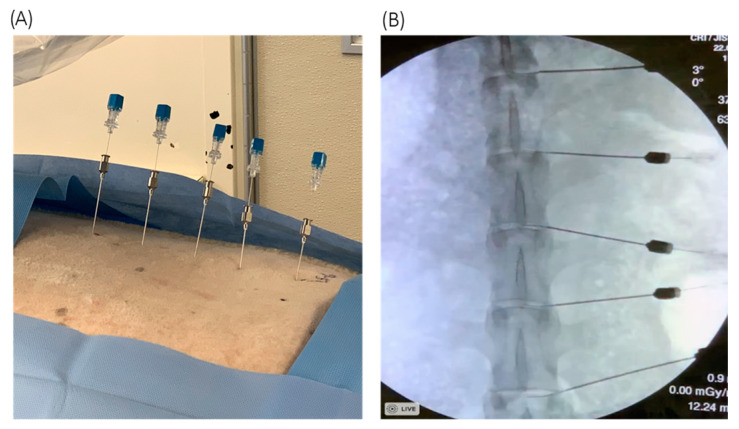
(A) 25-gauge needles within the 20-gauge introducer needles, serially spaced into the lumbar discs. (B) Fluoroscopic radiograph verifying needle placement in the AP plane.
